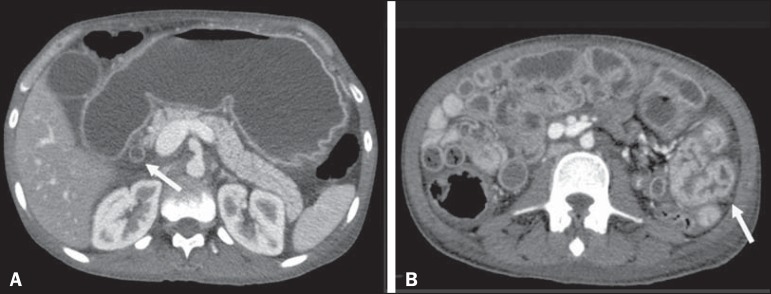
Intravenous contrast-enhanced CT scan showing accentuated gastric distension
